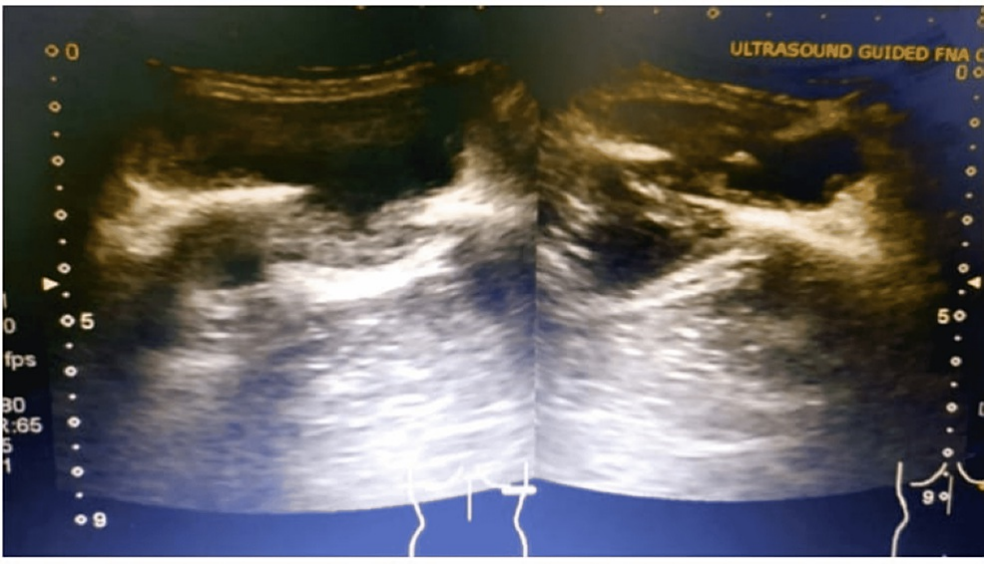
Ultrasound-guided aspiration of the right rib cage collection.
